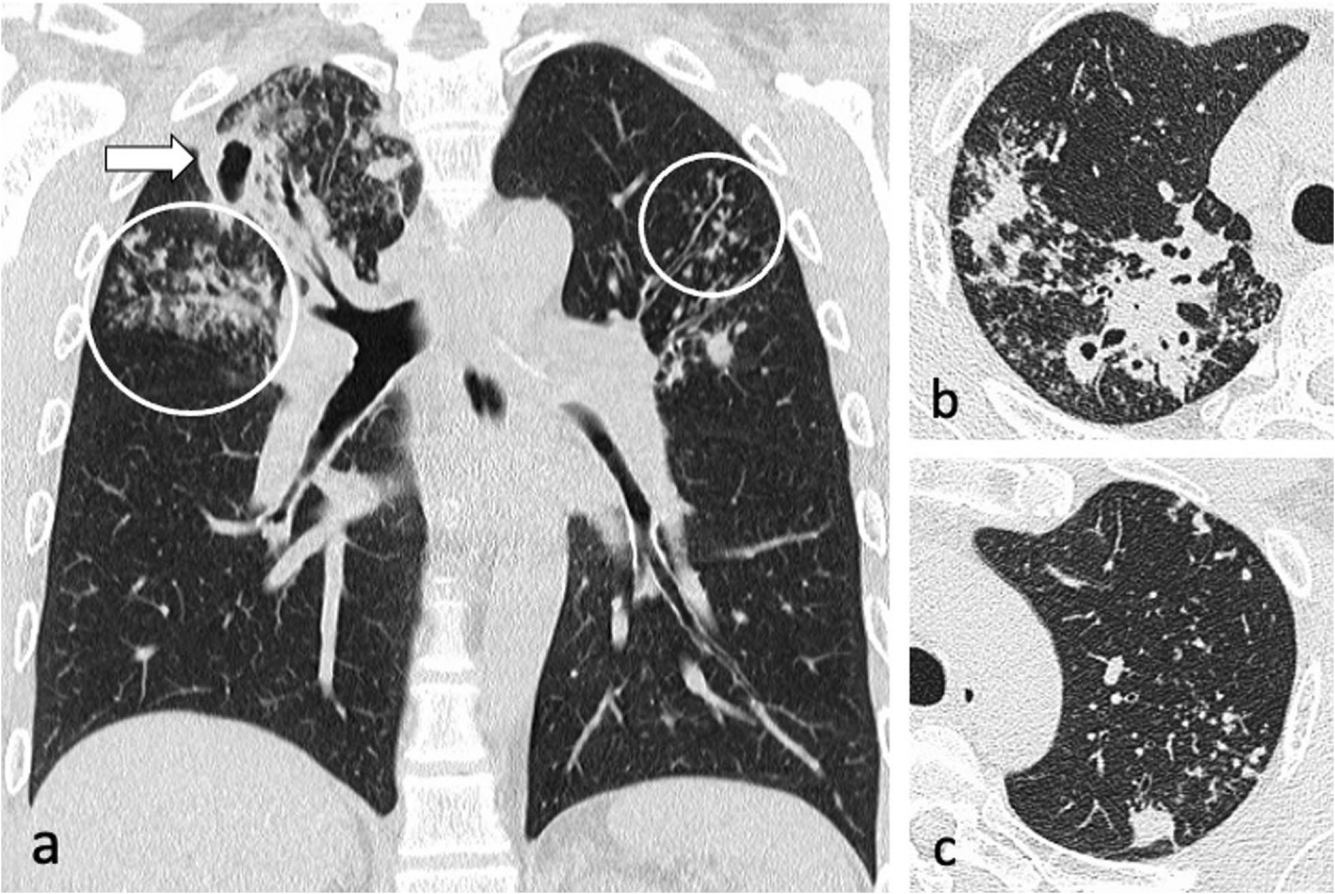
A 41-year-old male presented with chronic cough, weight loss and fevers. CT (lung window) demonstrates bilateral areas of centrilobular and tree-in-bud nodules (coronal plane—a, circled; axial plane—c) and consolidation in the right upper lobe (axial plane—b). There are also cavitating lesions with thick walls (arrow, a)
